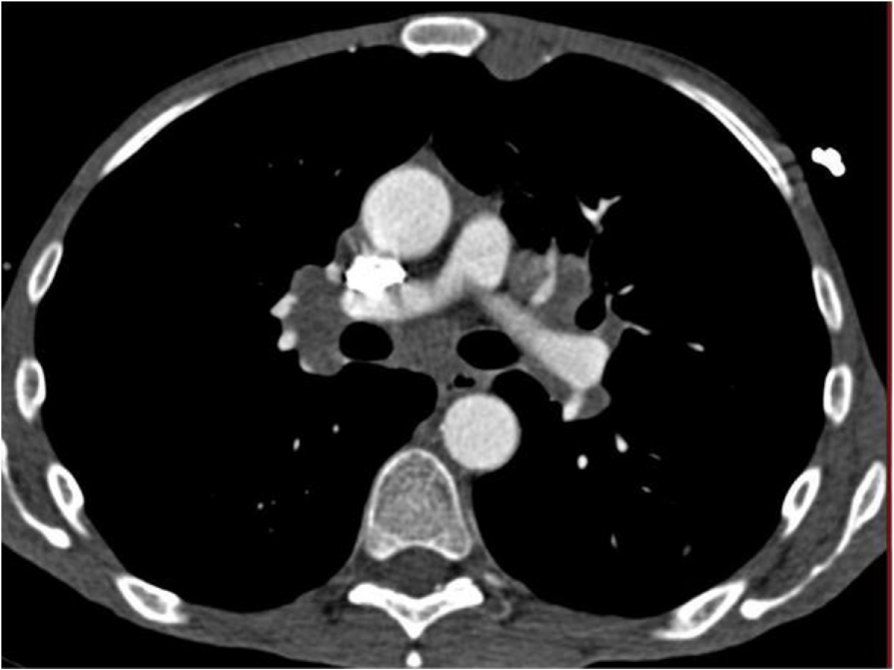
CT image of the thorax shows mediastinal lymph nodes.
